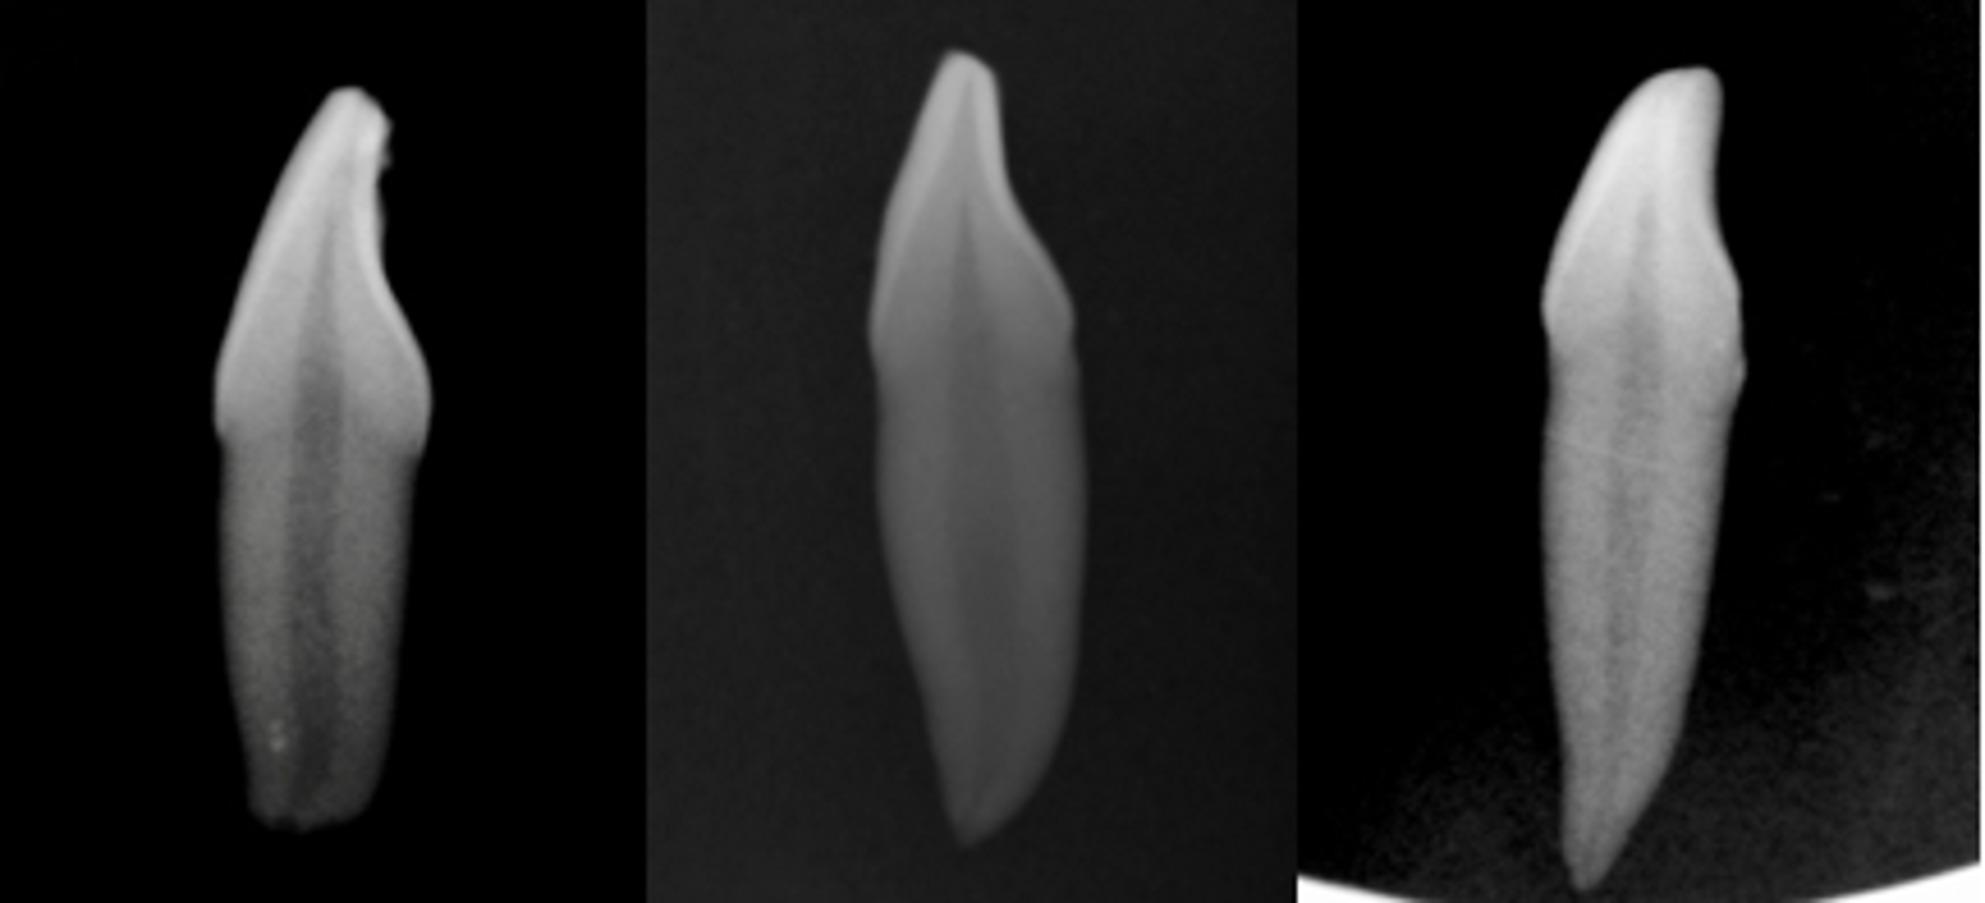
Periapical radiographs taken from extracted lower incisors
